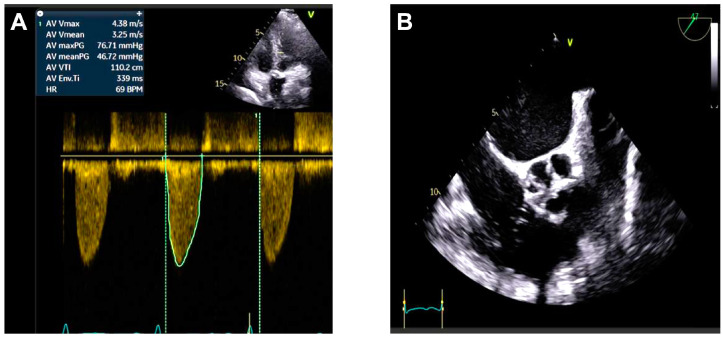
(A) Transthoracic echocardiography (TTE) images of the patient: the maximum peak gradient (AV maxPG) across the AV was 76.71 mmHg, the maximum systolic flow velocity (AV Vmax) was 4.38 m/s, and the mean gradient (AV mean PG) was 46.72 mmHg. (B) Transesophageal echocardiography showing a severely calcified aortic valve, suggestive of severe aortic stenosis.
